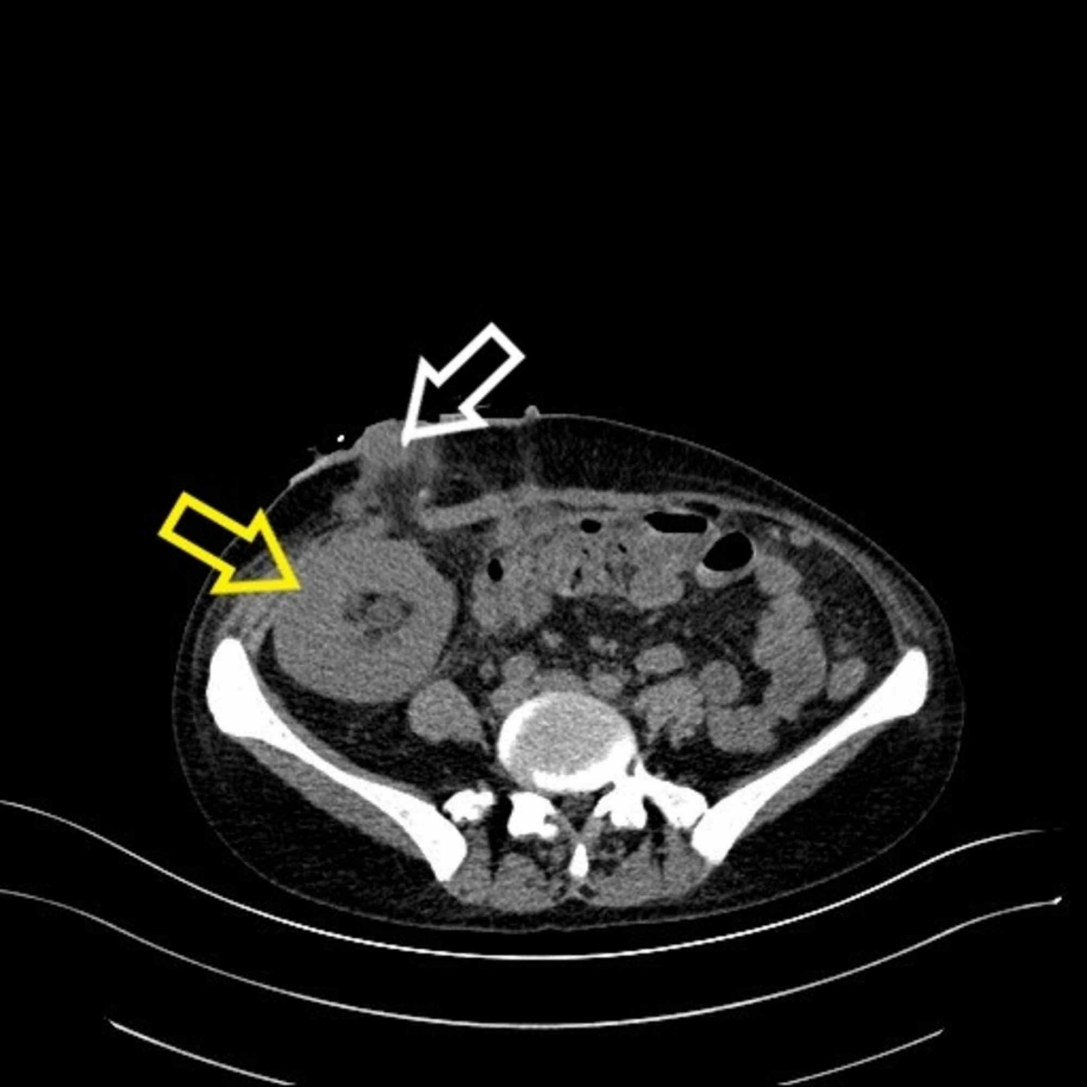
Non-contrast abdominal CT scan shows the transplanted kidney in the right iliac fossa (yellow arrow) and ileal conduit stoma (white arrow). CT, computed tomography
